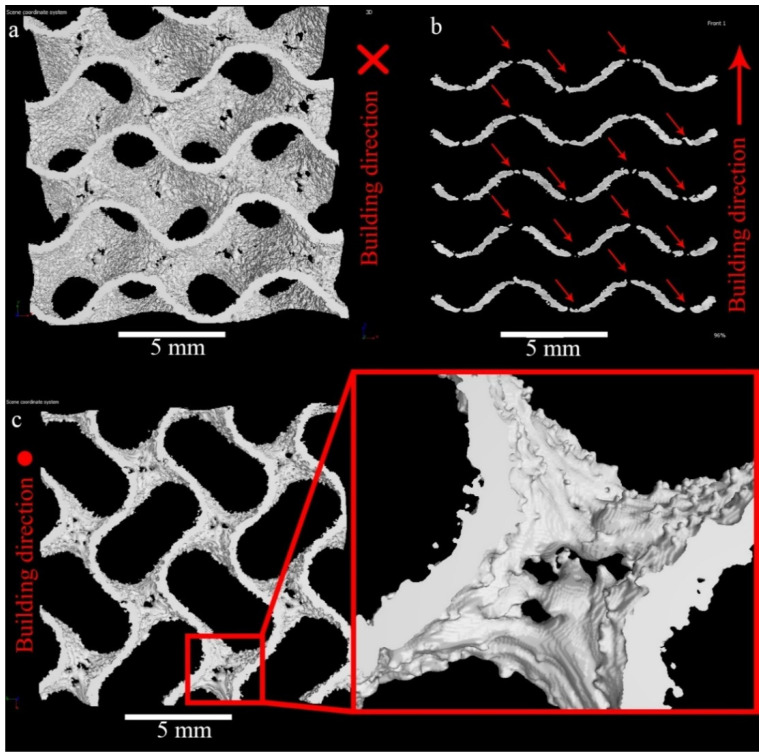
3D rendering of CT-reconstruction of WT gyroid vertical (a,c) and horizontal (b) views (a—bottom view; c—top view; b—side view).
